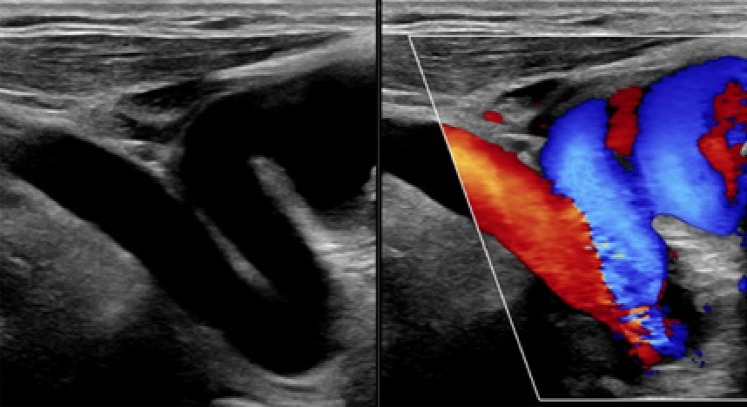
Doppler arterial de artérias carótidas e vertebrais esquerdas, mostrando um kink em origem de carótida comum.
